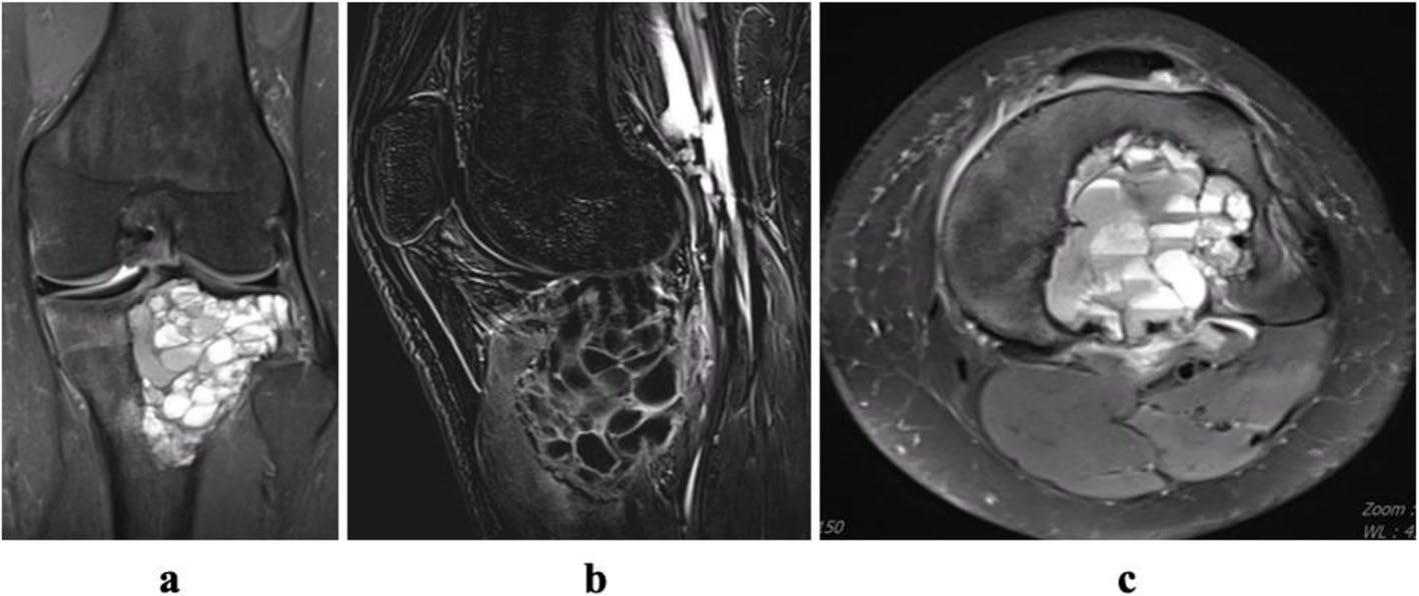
a Coronal MRI image showing fluid–fluid level. b Sagittal MRI image showing septa formation. c Axial MRI image showing the level difference
